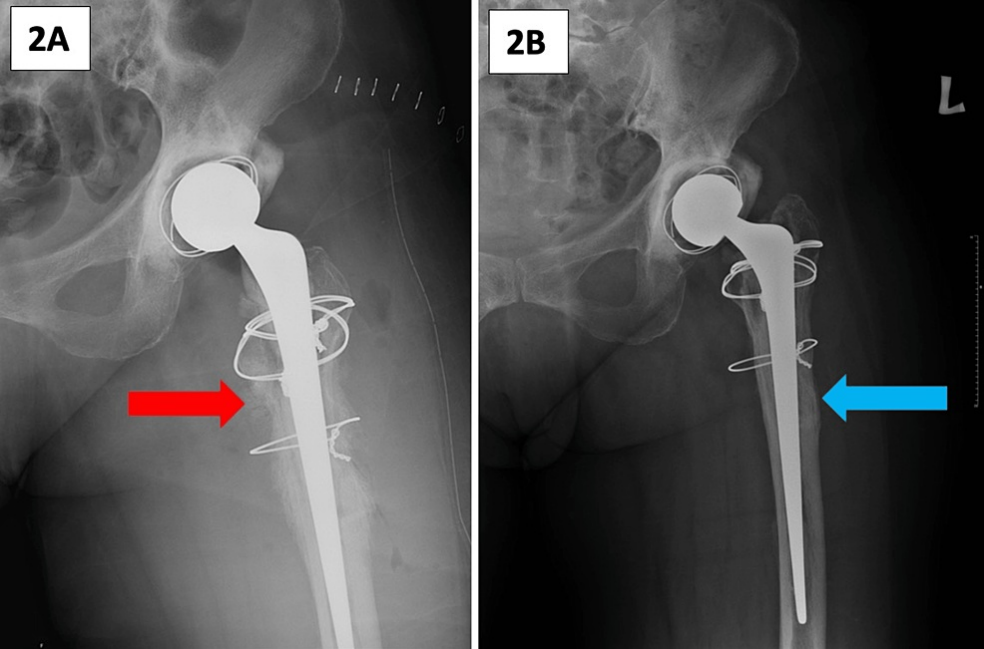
Left hip radiograph immediately post-surgery showing that the periosteal reaction (red arrow) is still present (A); left hip radiograph at one year post-surgery showing remodeling of the proximal femur (blue arrow) (B).
